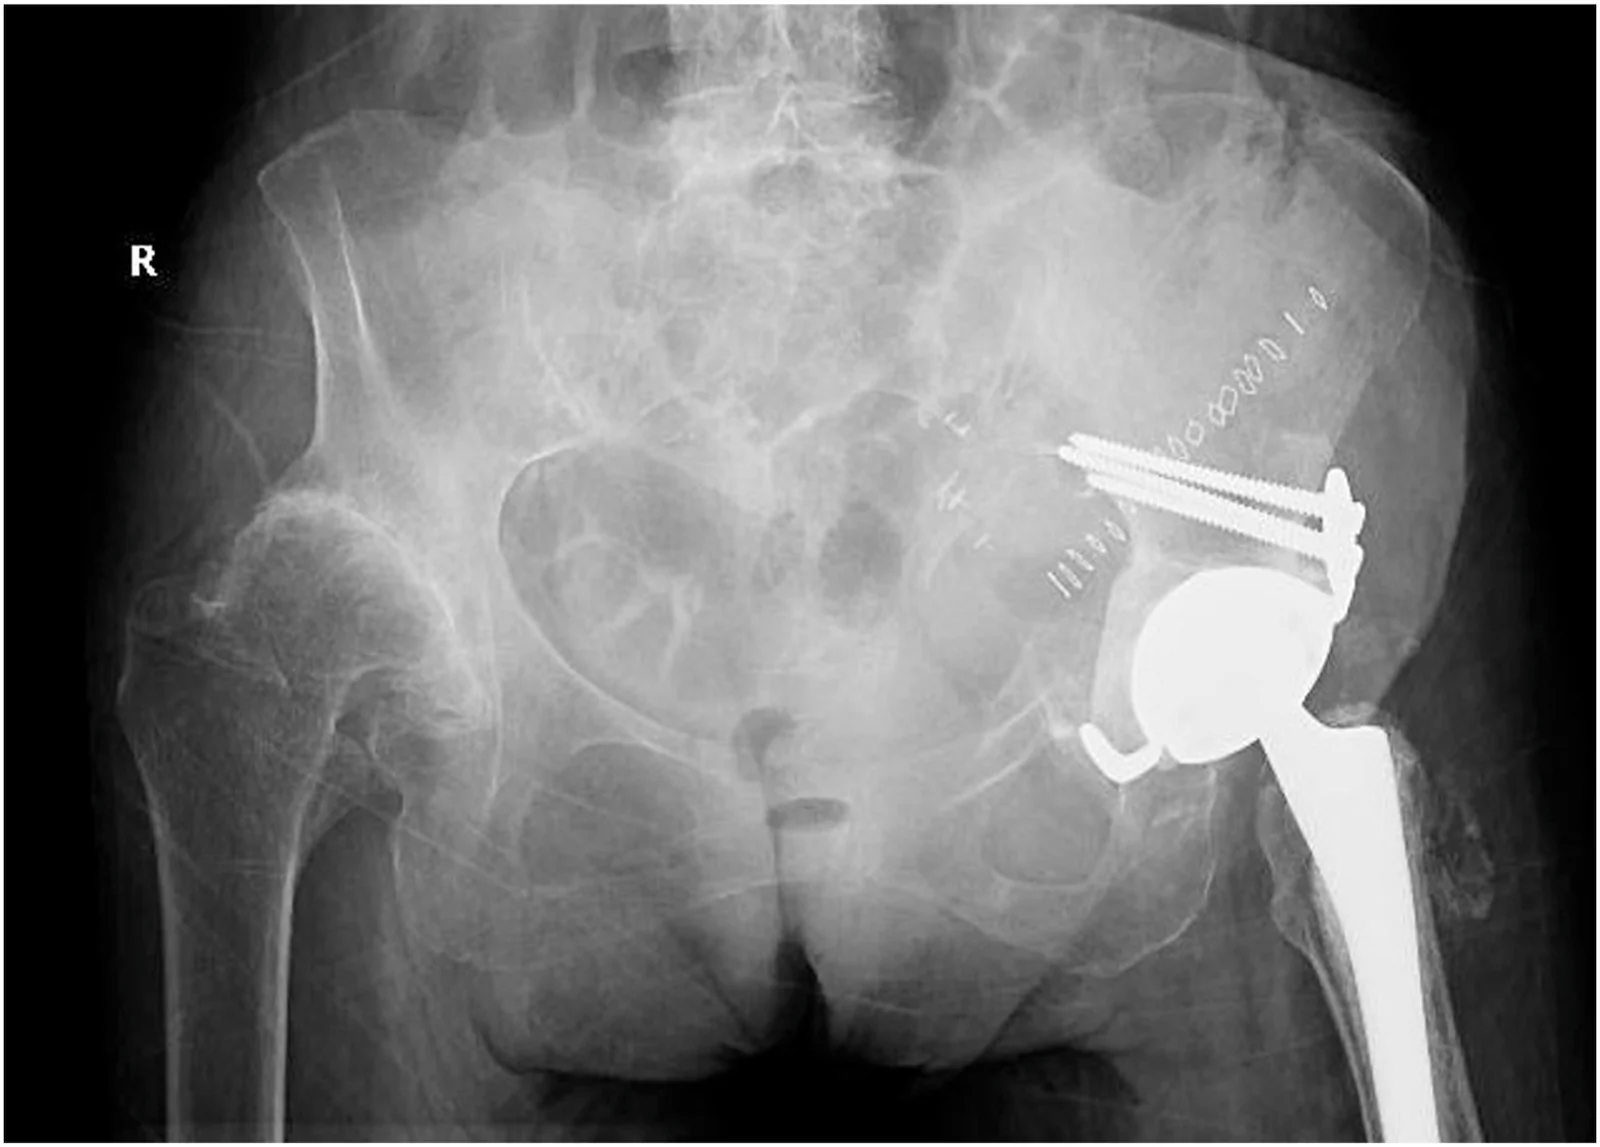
Final result. Anteroposterior pelvic radiographs after intrapelvic hardware removal.
